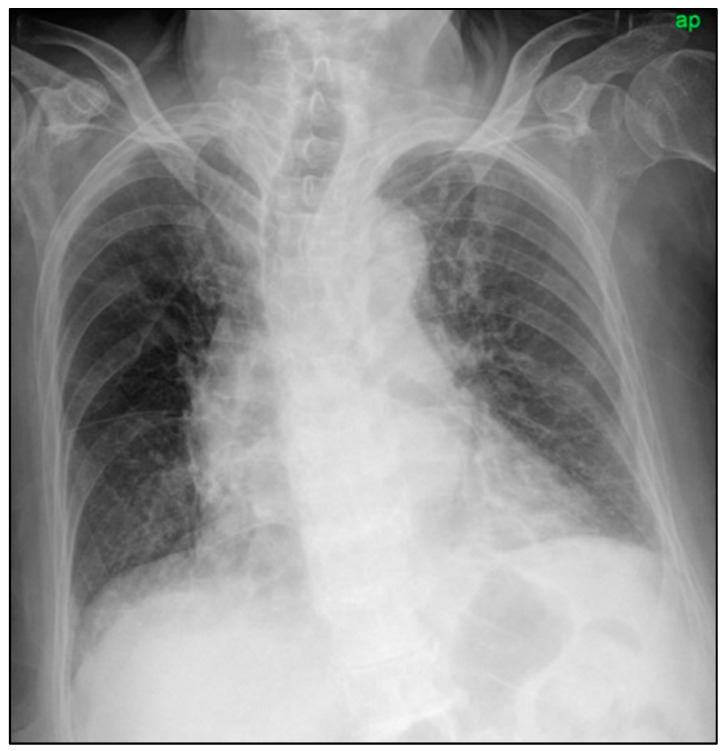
Supine anteroposterior chest radiograph showing suspicious infiltrates in the right upper lobe and both lower lobes.
